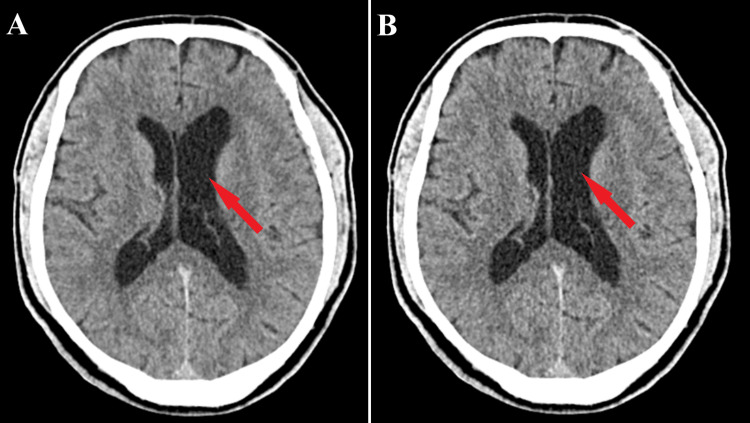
Follow-up CT scan The axial section of the CT scan at three-month follow-up (A) and axial section of the CT scan at six-month follow-up (B) both show no residual tumor or recurrence (red arrows) with a slightly enlarged left lateral ventricle
